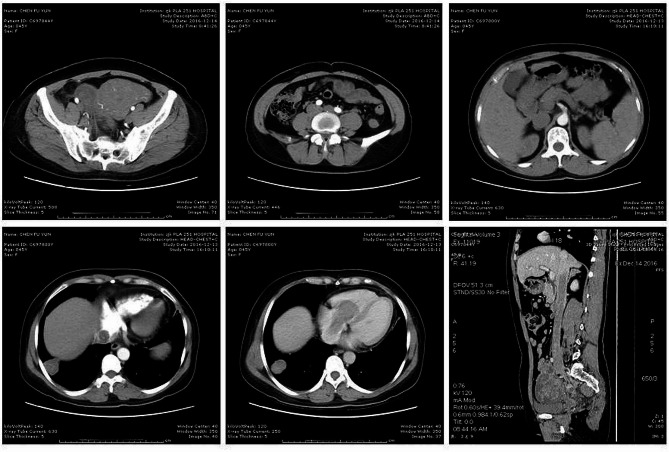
Computed tomography (CT) of pelvic cavity and heart identified a 13.7 × 5.4 cm [2] sized, irregular shaped, unclear boundary, and soft tissue density mass within the uterus. The tumor extended through inferior vena cava into the right atrium and ventricle
